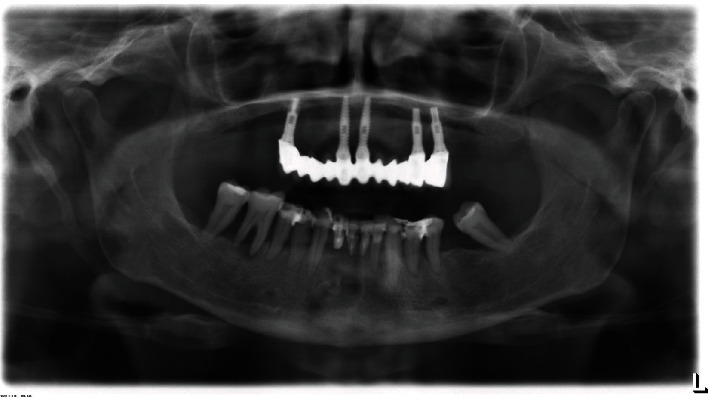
Orthopantomography performed in 2019.
